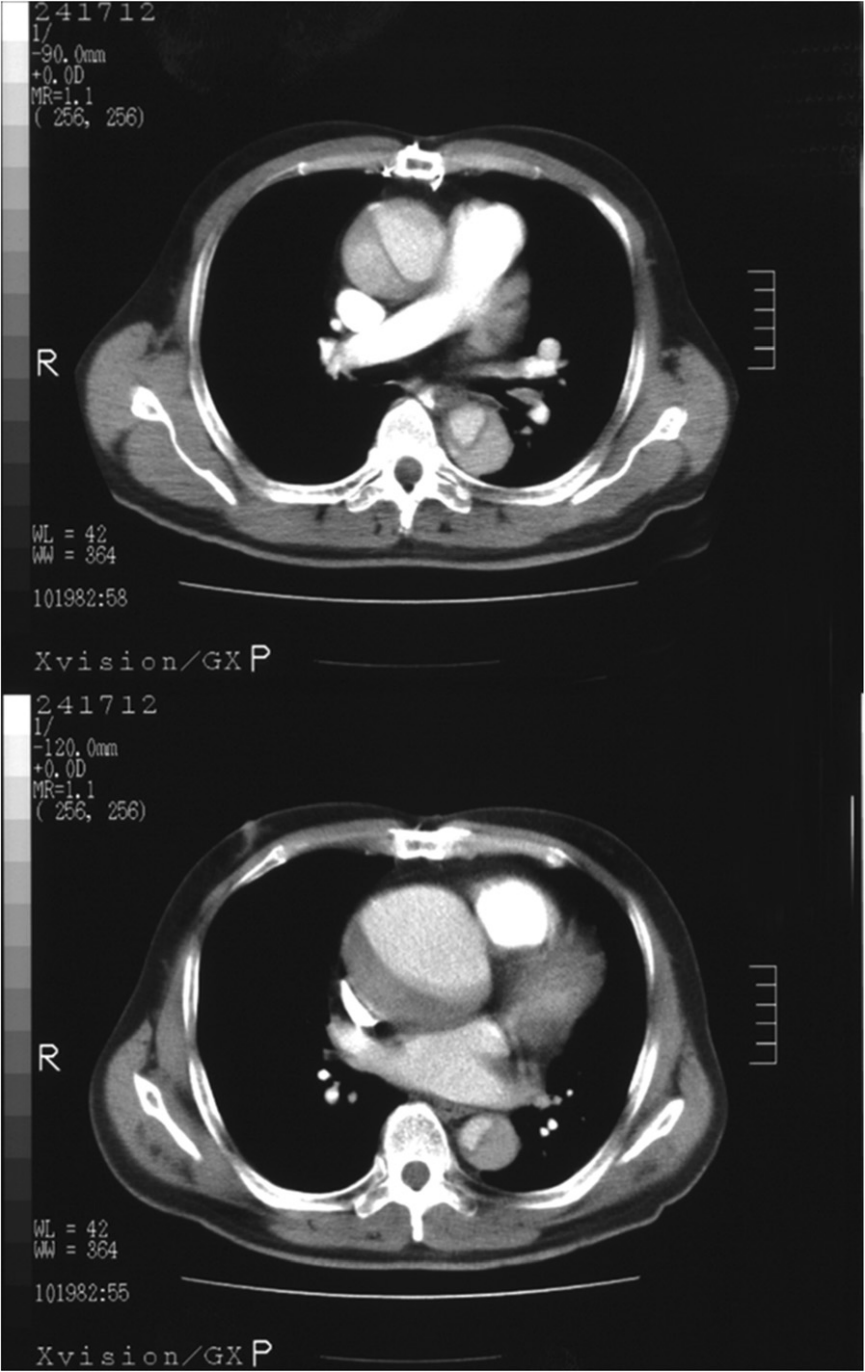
Computed tomography angiography (CTA) showing dissection of the ascending aorta.
